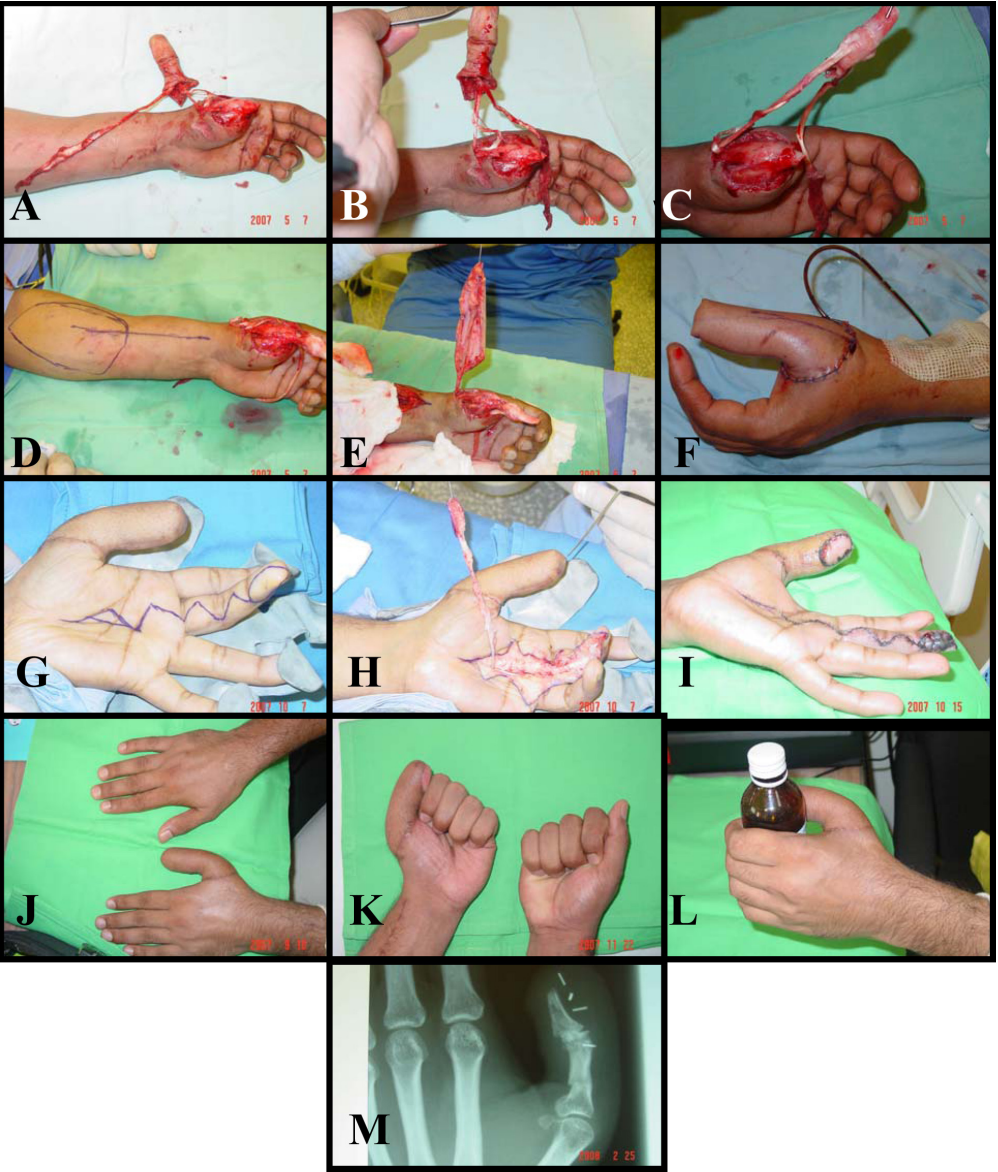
A, B crush avulsion injury. C: Skelotinize the bone. D: Marking the radial forearm flap & fixing the bone graft by two crossing K wires. E, F: Raising & setting the flap. G, H, I: Marking raising & setting the sensate island (Littler flap). J, K, L: final result M: X-ray shows bone healing.
